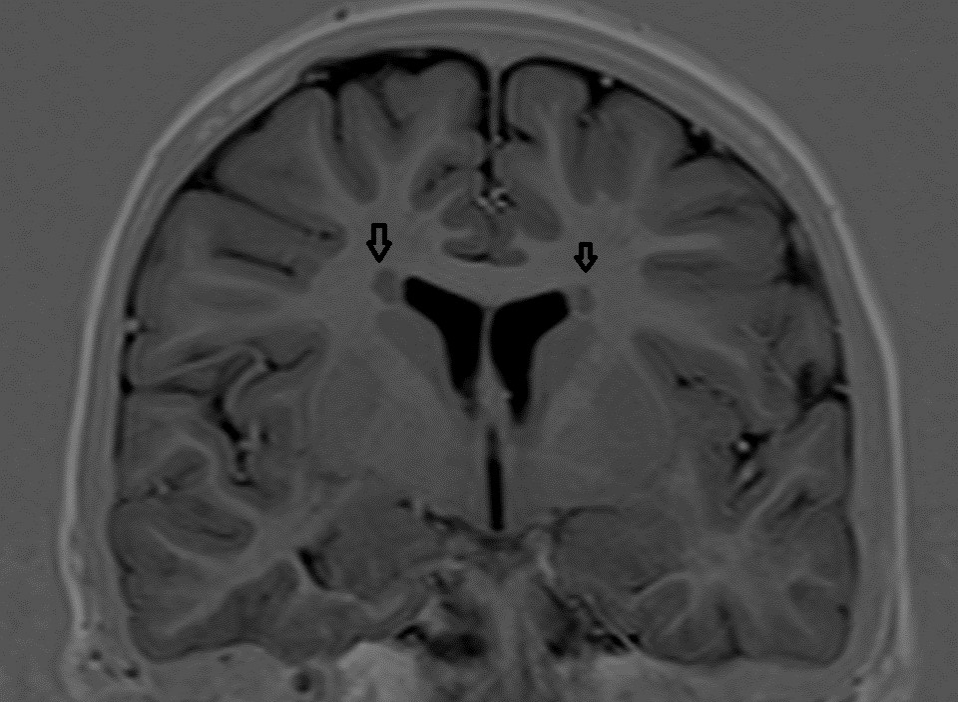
Brain magnetic resonance imaging in a patient with a novel *KMT5B* variant (coronal section): periventricular nodular heterotopia marked with arrows.
